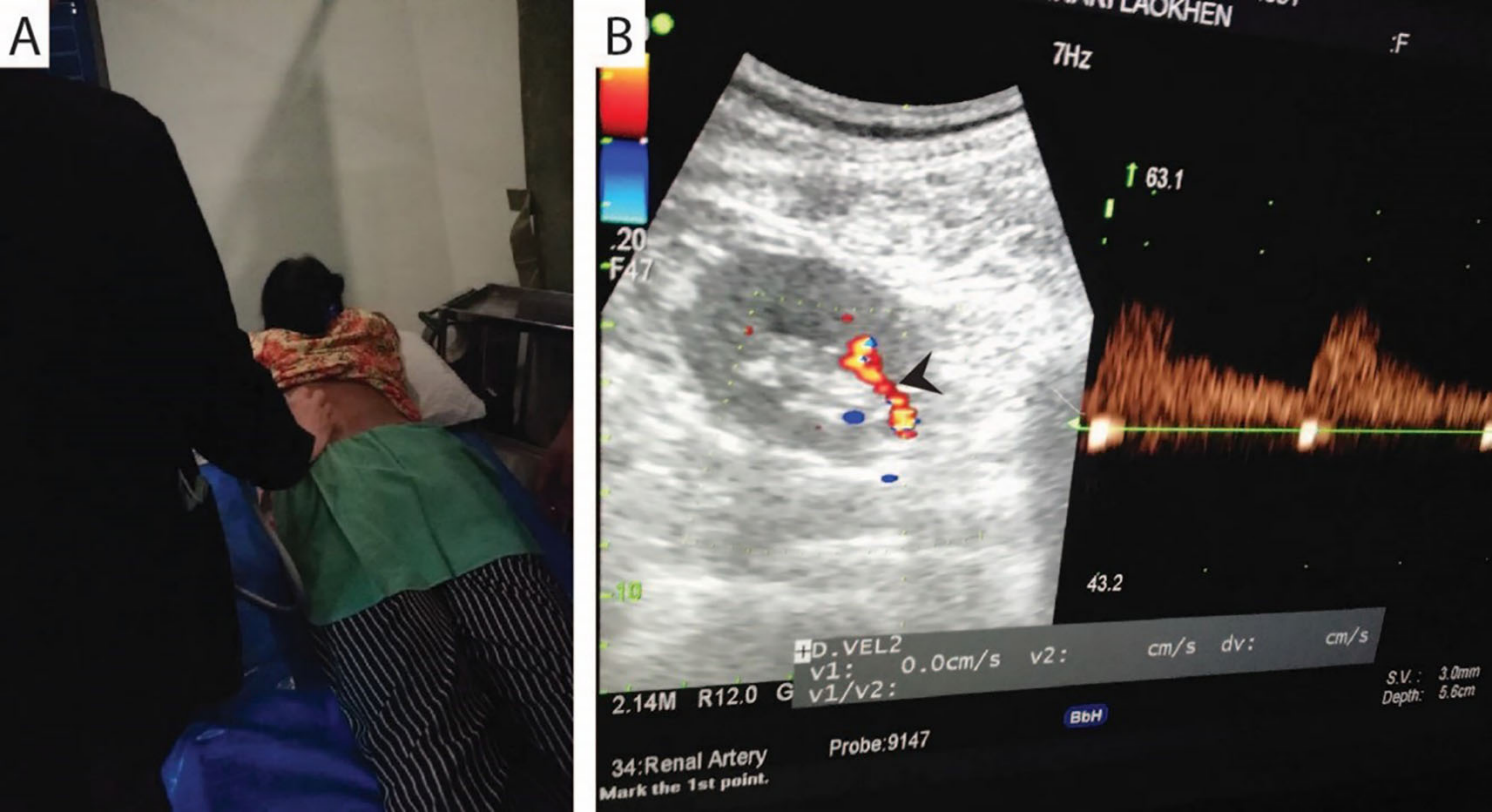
Examination of renal blood flow by a radiologist using Doppler ultrasound (A). Color flow ultrasound image demonstrates a representative left renal artery (B, arrowhead).
